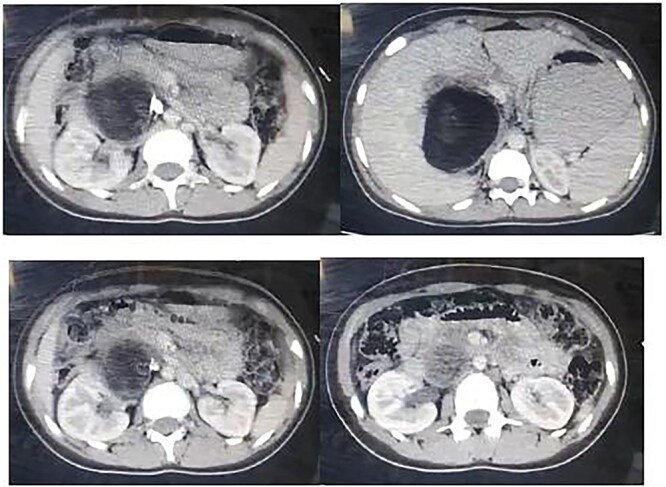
Non contrast CT showing a large hypogenous cyst of the caudate liver lobe lengthen to the hepatic hilum, dimensions of 7.7 × 9.5 cm with calcified composition of the wall.
